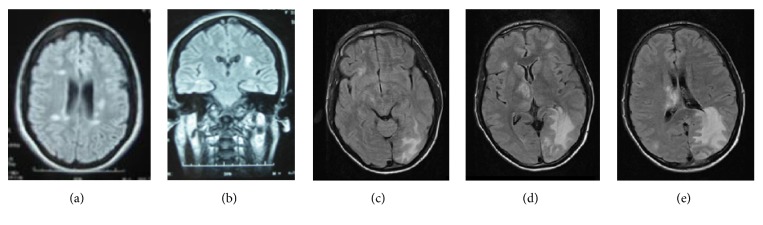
Brain MRI showed demyelinating lesions in a patient with multiple sclerosis (a, b) [S1] and haemorrhagic leukoencephalopathy due to toxoplasmosis in HIV patient (c, d, e) [S3].
